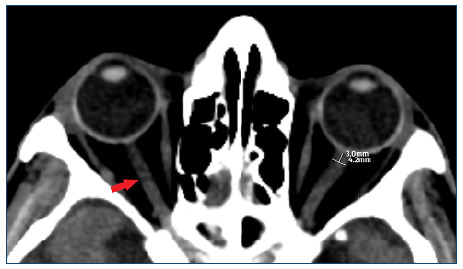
In the axial computed tomography section, the right optic nerves (red arrow) (a) and the location where the left optic nerve sheath diameter measurement was done (b).
